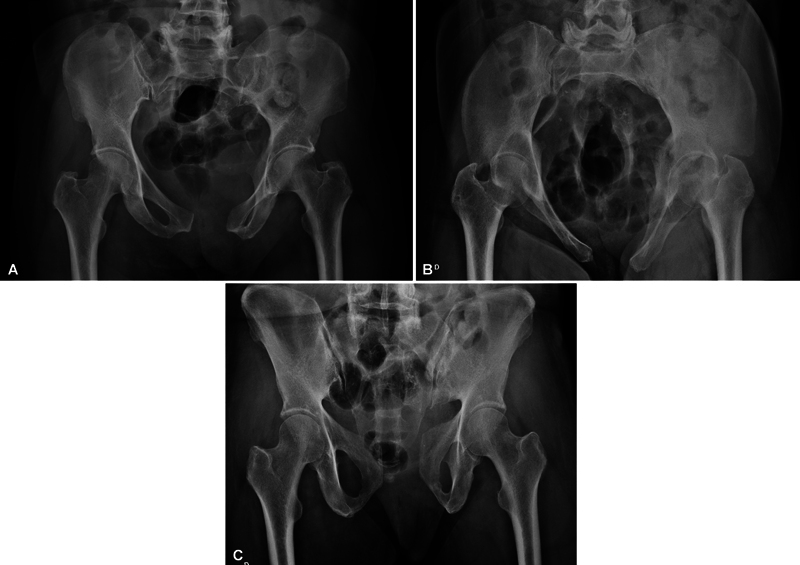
Case example (preoperative radiographs). Anteroposterior (AP) (A), inlet (B), and outlet (C) radiographs of a female patient presenting a mechanically unstable pelvic ring injury, including a complete and nondisplaced sacral fracture on the right side, incomplete injury of the left sacroiliac joint, and a pubic symphysis injury.
